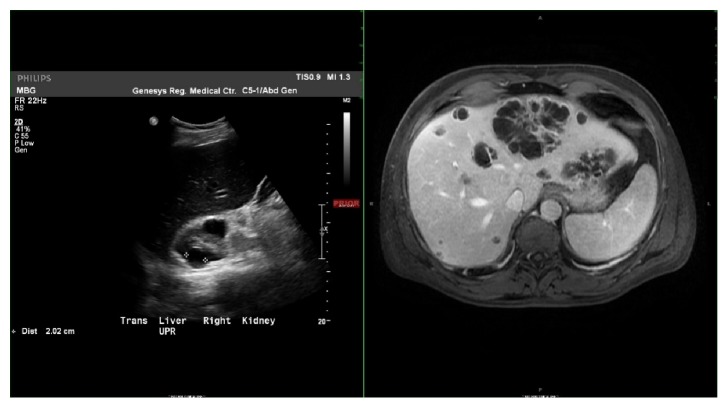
Transverse imaging showing initial negative ultrasound (left) and follow-up MRCP showing liver abscesses (right).
